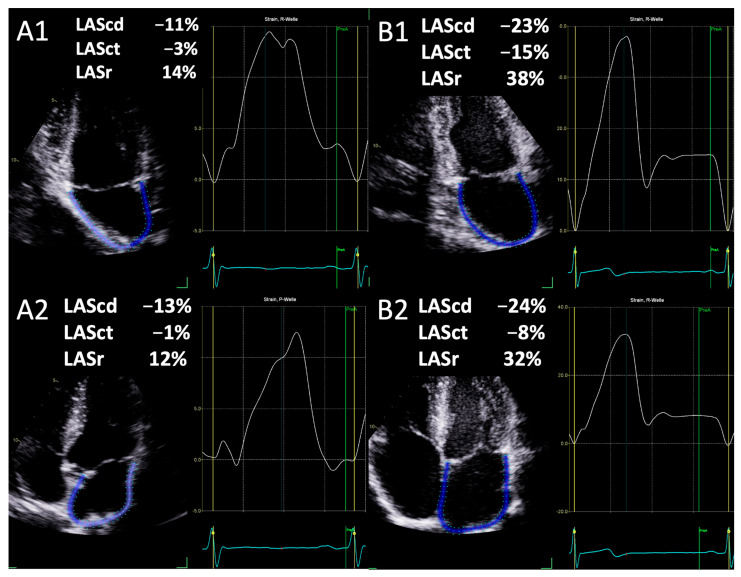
Assessment of left atrial conduction strain (LAScd), contraction strain (LASct), and reservoir strain (LASr) in apical 2-chamber view (A1,B1) and 4-chamber view (A2,B2) in upright posture (A1,A2) and left lateral position (B1,B2).
